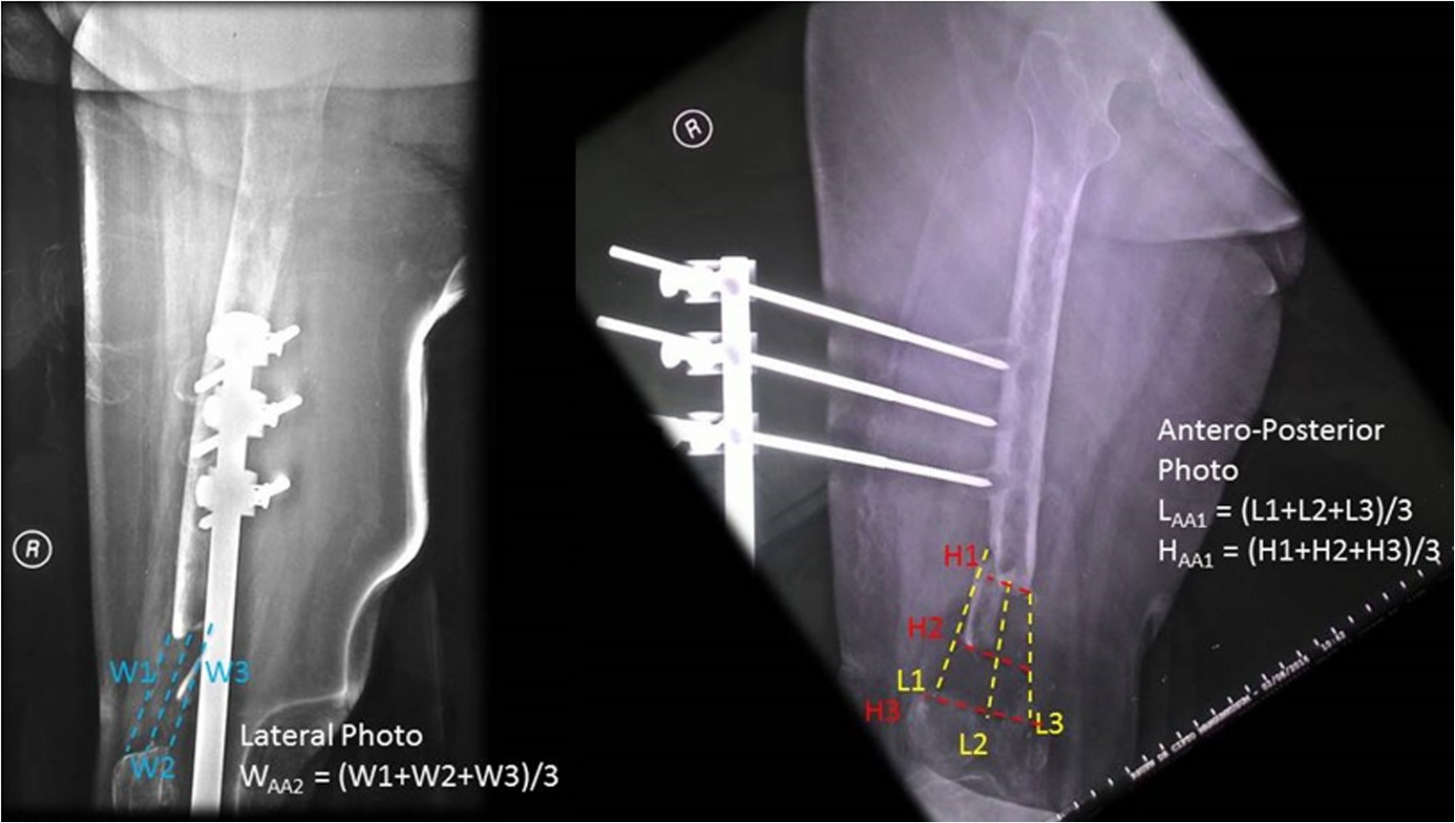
Volume defect measurement by means of radiography
